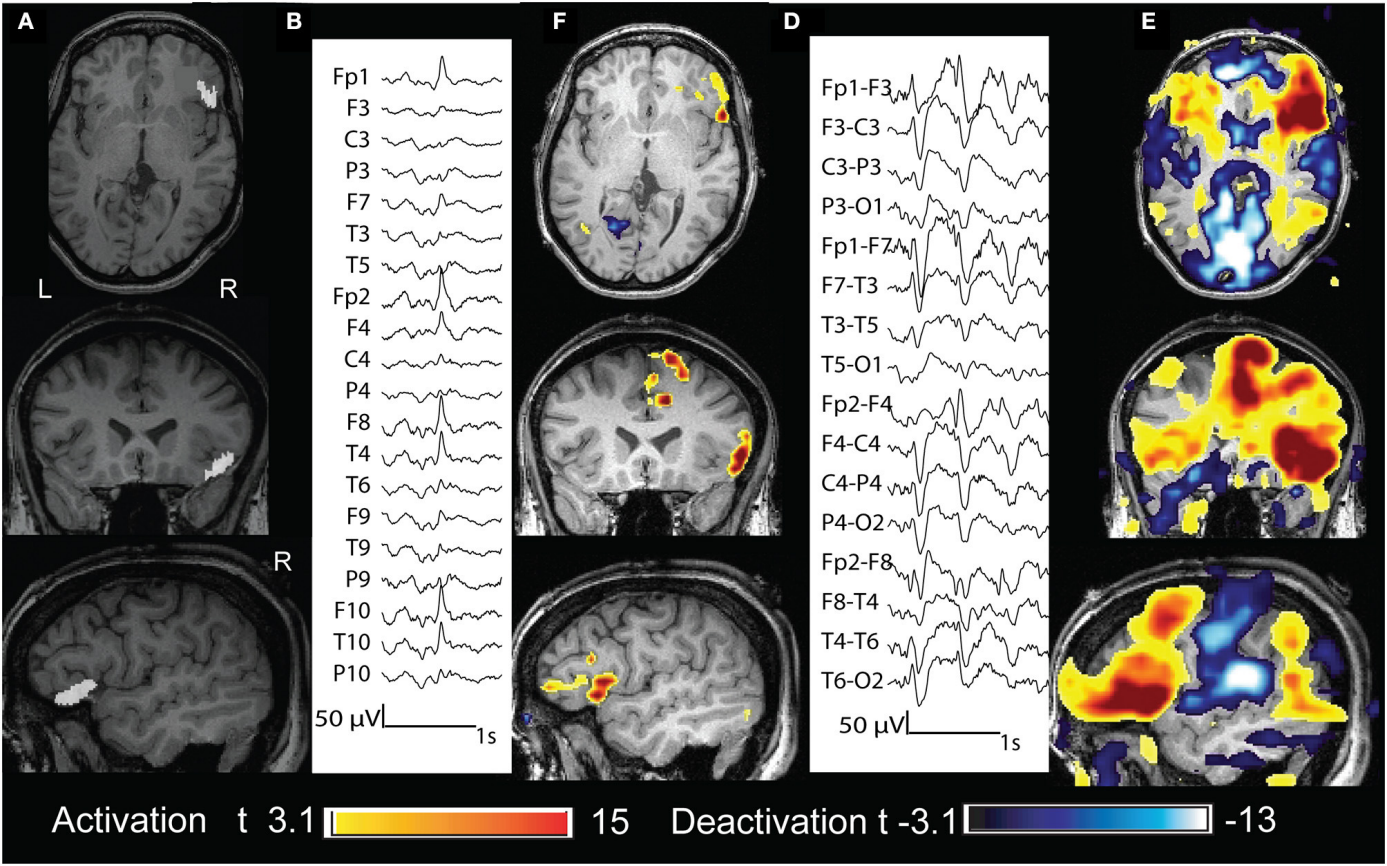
Twenty three year old patient (case 4). (A) Structural MRI shows a thickening of the cortical gray matter and blurring of the gray-white matter interface in the right frontal operculum (in white). (B) The marked events were spikes with maximum amplitude at Fp2 F8 F10 (reference Fz). (C) BOLD response was characterized by different clusters of activation. That with max t value is in the right frontal operculum, corresponding to the lesion. (D) The marked events were burst of spike and wave complexes bilateral and diffuse (bipolar montage), but more evident on the right fronto temporal region. (E) BOLD response was characterized by different clusters of activation: those with the highest *t*-values were in the right frontal operculum, in the right mid cingulate cortex and cerebellum; clusters of deactivation were present in the areas of the DMN.
